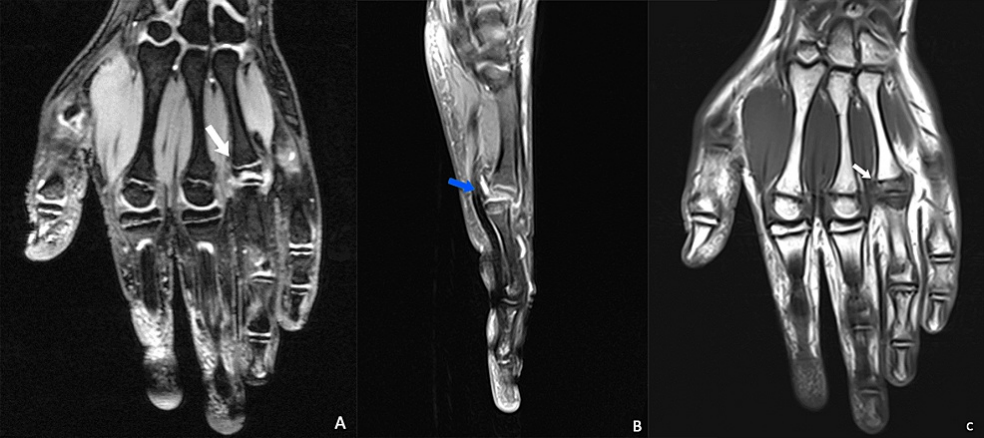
MRI imaging of the left hand and wrist (a) Coronal PD-FS and (b) sagittal T2 weighted images of the left hand depict subchondral marrow edema in the fourth metacarpal head (white arrow), with minimal joint fluid and volar displacement of the proximal fourth phalanx over the abnormal metacarpal head (blue arrow). (c) Coronal T1 weighted image of the left hand shows flattening and irregularity of the fourth metacarpal head with hypointense marrow signal in the subchondral bone (white arrow) MRI: magnetic resonance imaging
